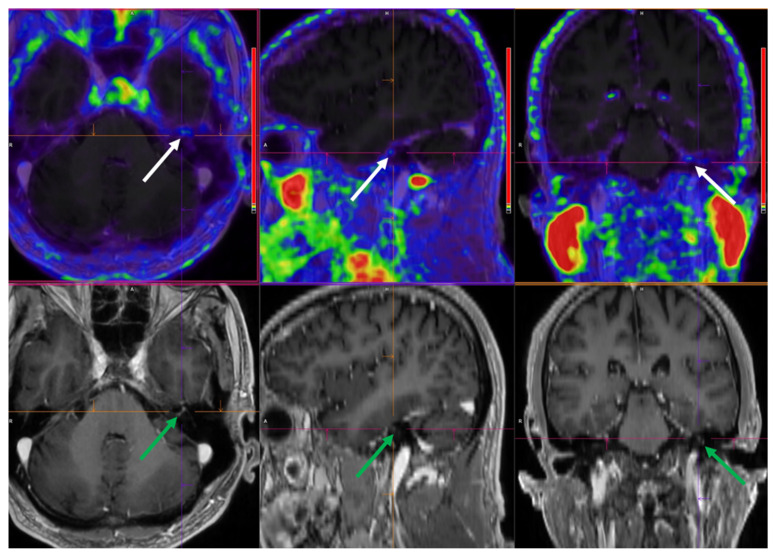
^64^Cu-DOTATATE PET fused with T1 post-contrast MRI in patient with neuroborreliosis. (Transaxial, sagittal and coronal view, respectively). Abbreviations: R = Right, H = Head, A = Anterior. Focal ^64^Cu-DOTATATE uptake in the left cranial facial nerve corresponds to MRI enhancement and left facial palsy symptoms. PET/CT images (upper rows) show discrete focal uptake of ^64^Cu-DOTATATE (SUV_max_ = 0.9) in the left cranial facial nerve (white arrows), corresponding to the contrast-enhanced segment observed on MRI (lower rows, green arrows).
